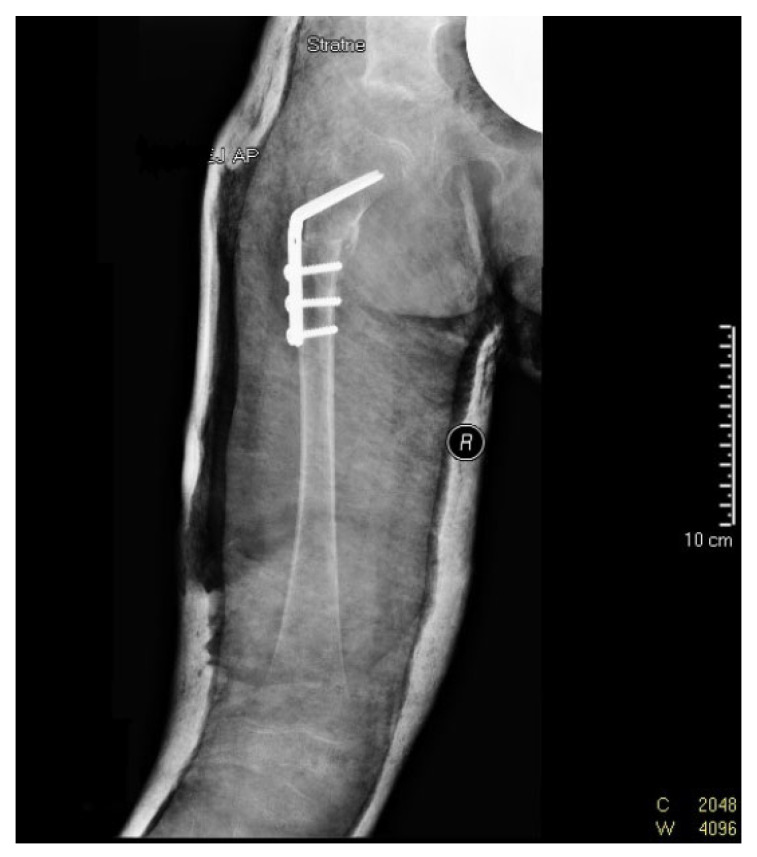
X-ray scan following varus and de-rotative osteotomy on the proximal part of the right femoral bone (at 6 years of age).
